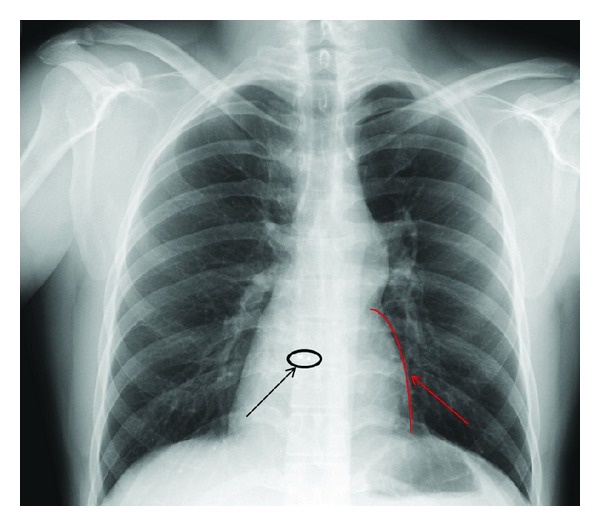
X-ray changes anticipated in ccTGA with right ventricular border outlined with red arrow and Amplatzer occluder device outlined with dark arrow.
